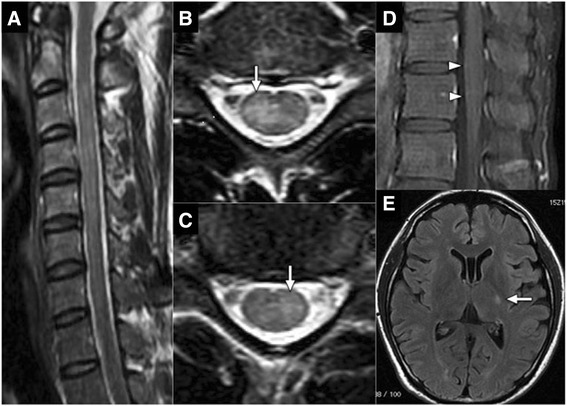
Spinal cord T2-weighted MRI shows a hyperintense lesion extending from C2 to C6 on the sagittal view (a), and predominantly in the central gray matter on the axial view at C4 (b. *arrow*) and C6 level (c. *arrow*). Gadolinium-enhanced T1-weighted MRI shows slight gadolinium enhancement within the conus medullaris surface (d. *arrow heads*). Brain fluid-attenuated inversion recovery MRI shows a hyperintense lesion in the left crus posterius capsulae internae (e. *arrow*)
